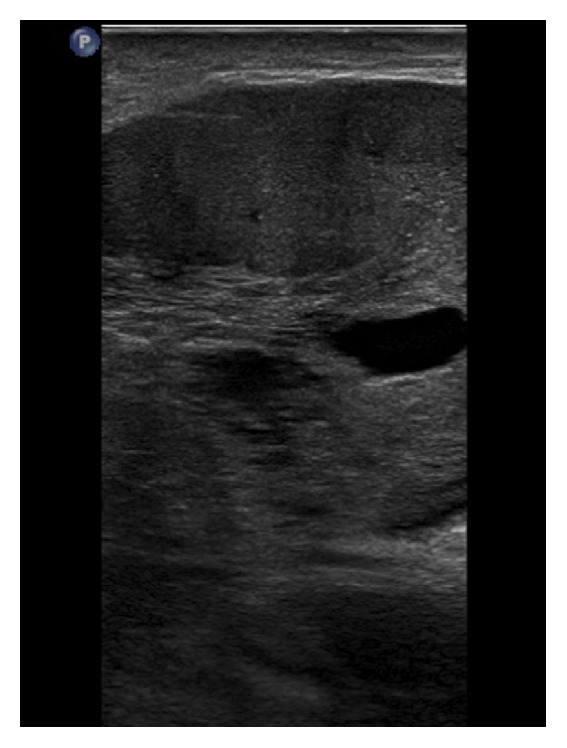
Sonography of the patient's left breast showing hypoechoic mass with solid appearance and multiple cystic changes.
